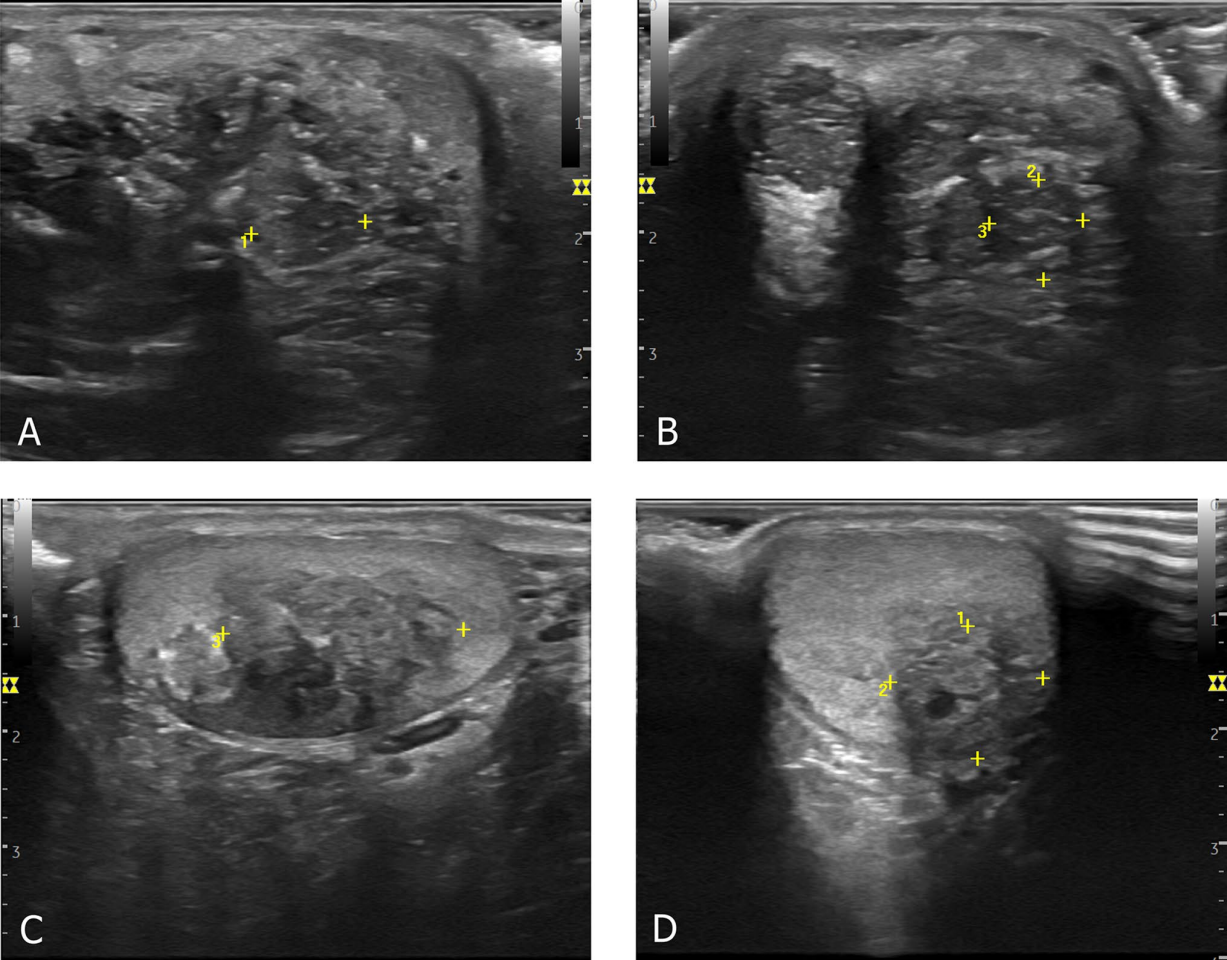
Ultrasound pictures of the testicular adrenal rest tumors. Upper panels: longitudinal (A) and transversal (B) scans of the right testis. Lower panels: longitudinal (C) and transversal (D) scans of the left testis
